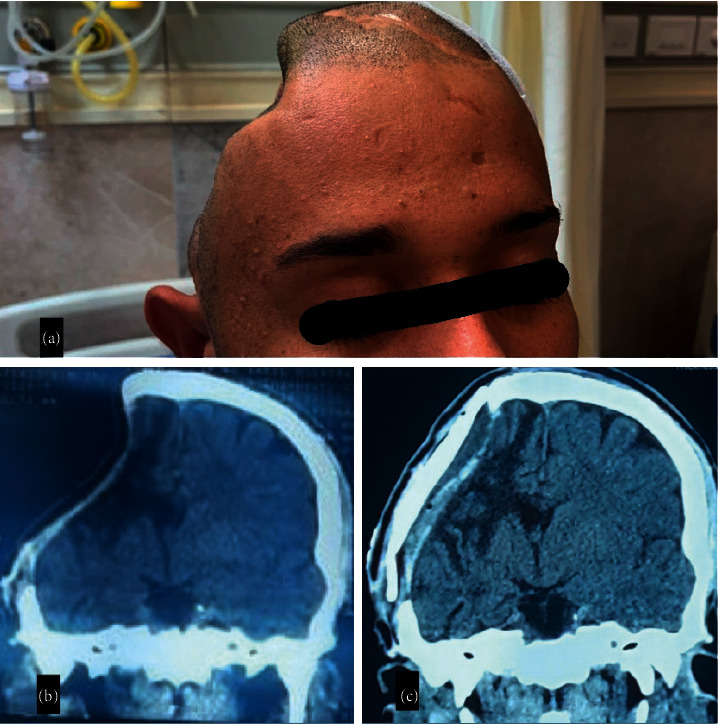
(a) Clinical photograph showing sunken skin flap 3 months after craniectomy. (b) Computed tomography (CT) plain brain, coronal view showing right-sided craniectomy with severely sunken hemisphere on the same side. (c) Computed tomography (CT) plain brain, coronal view on postoperative day 1, showing cranioplasty using flattened bone. Also, partial resolution of pressure effects can be seen.
